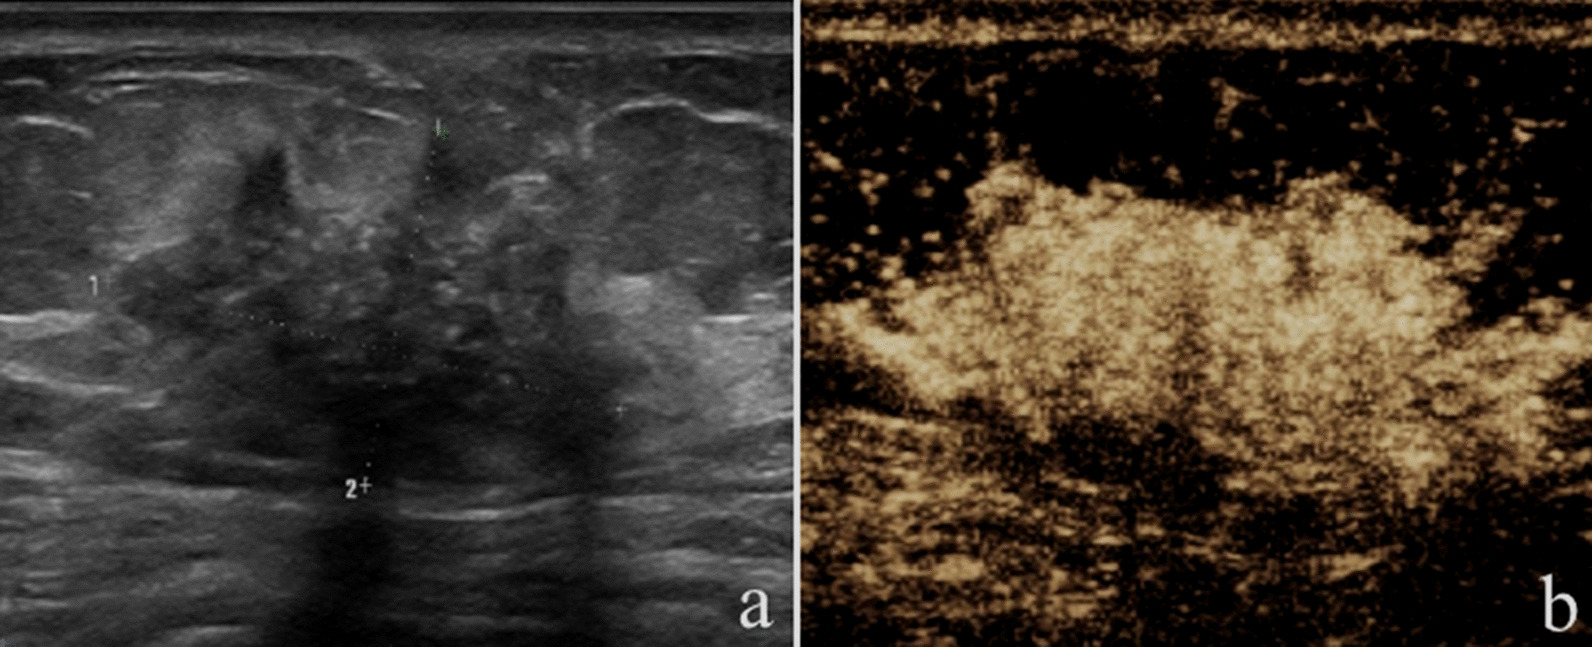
Image of a 38-year-old woman with a nonpalpable mass. a B-mode ultrasound image; b contrast-enhanced ultrasound image showed that the lesion displayed hyper-enhancement with ill-defined enhancement margin and enlarged size on CEUS. The CEUS score was 4 points. This lesion was originally classified as US BI-RADS 4C, upgraded to BI-RADS 5 after CEUS, and both were diagnosed as atypical ductal hyperplasia at US-guided core needle biopsy and at surgery
